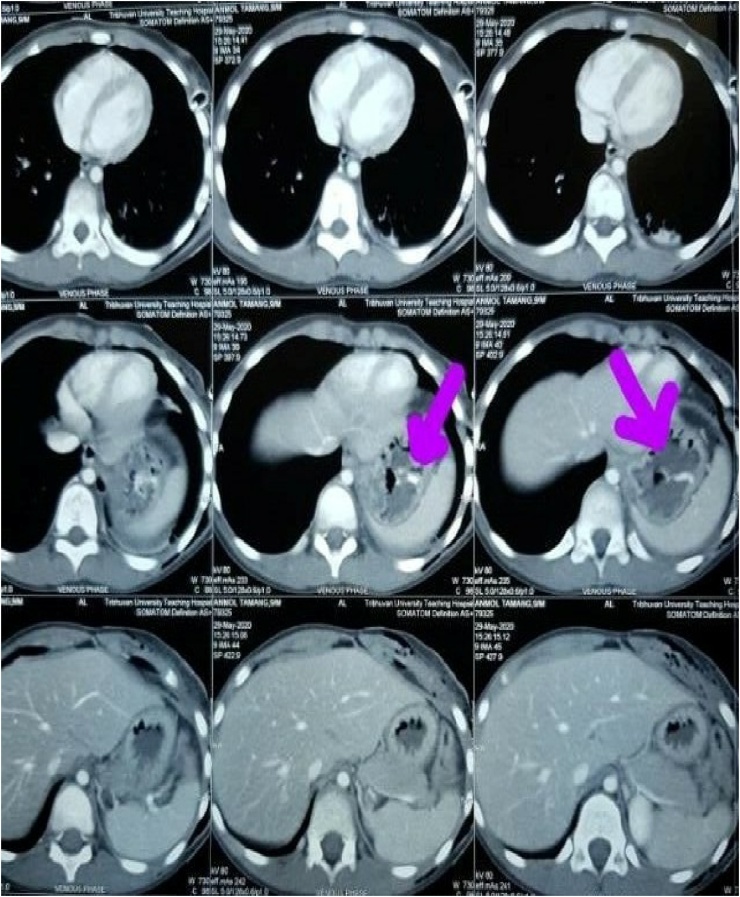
Preoperative CT scan show perforations.
